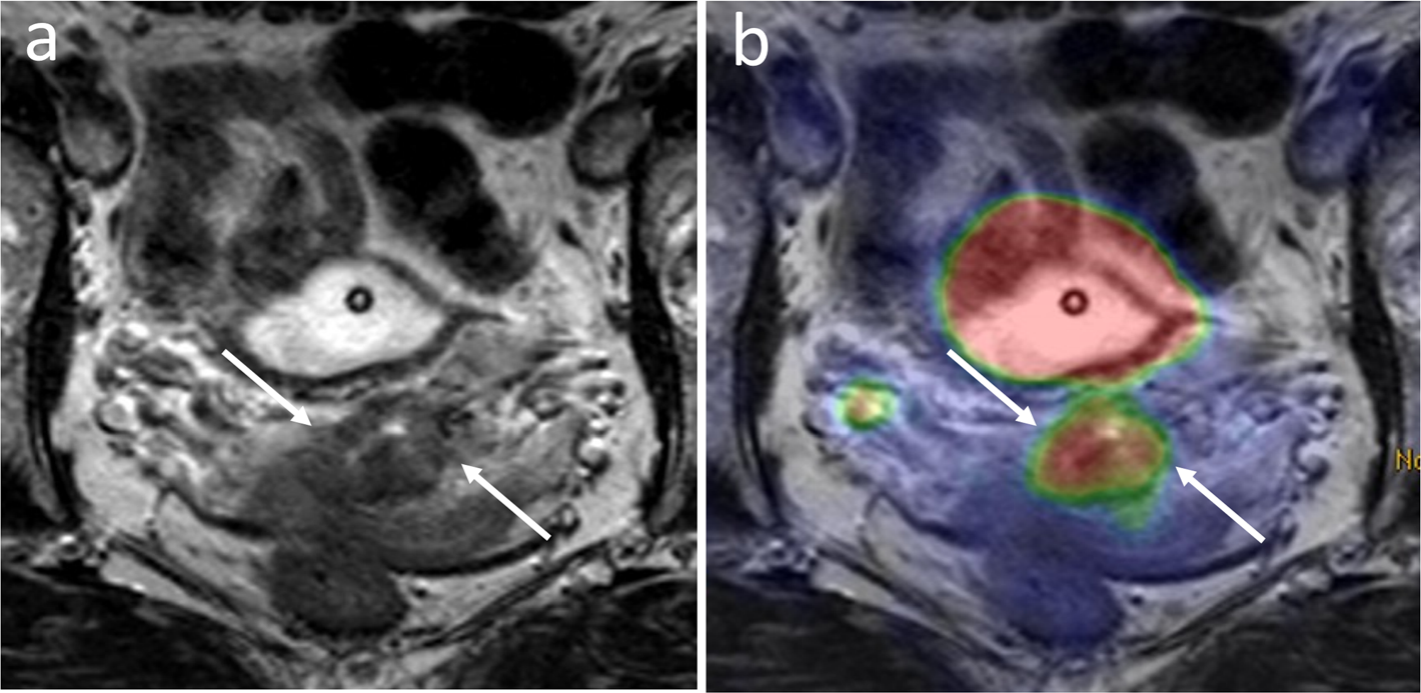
A 48-year old woman with stage T1b1 cervical cancer that was misinterpreted as stage T2b on MRI. Axial T2-weighted image shows the cervical cancer with some irregularity of the stromal rim (a, white arrows) interpreted as parametrial invasion by MRI. PET/MRI shows the tumor confined to the cervix with no FDG uptake in areas of stromal irregularities (b). The patient underwent surgical staging including parametrial biopsies and sentinel node dissection with ultrastaging, followed by curative chemoradiation (patient no. 15 on Table 2)
